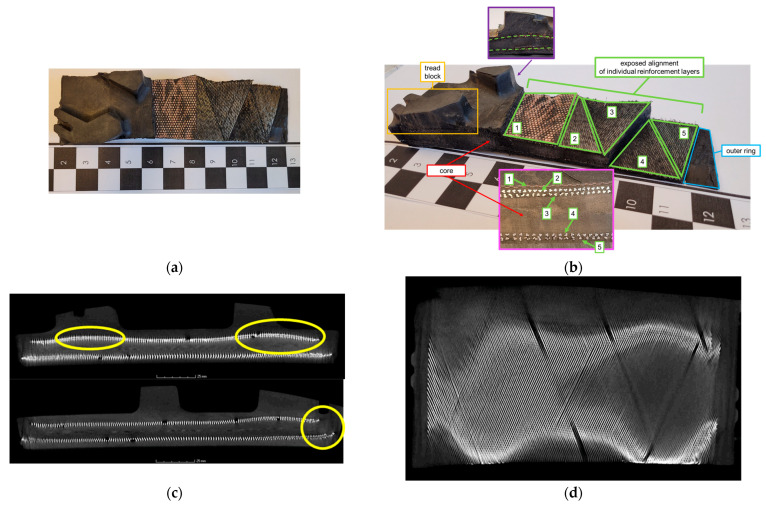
Portion of shear beam—NPT with 2D layered structure: (a) view from the tread side with cut-outs, (b) identification: reinforcement layers; tread block; outer ring, core, (c) reinforcement layers (micro-computed tomography), (d) arrangement of the “threads” in the reinforcement layers (micro-computed tomography).
